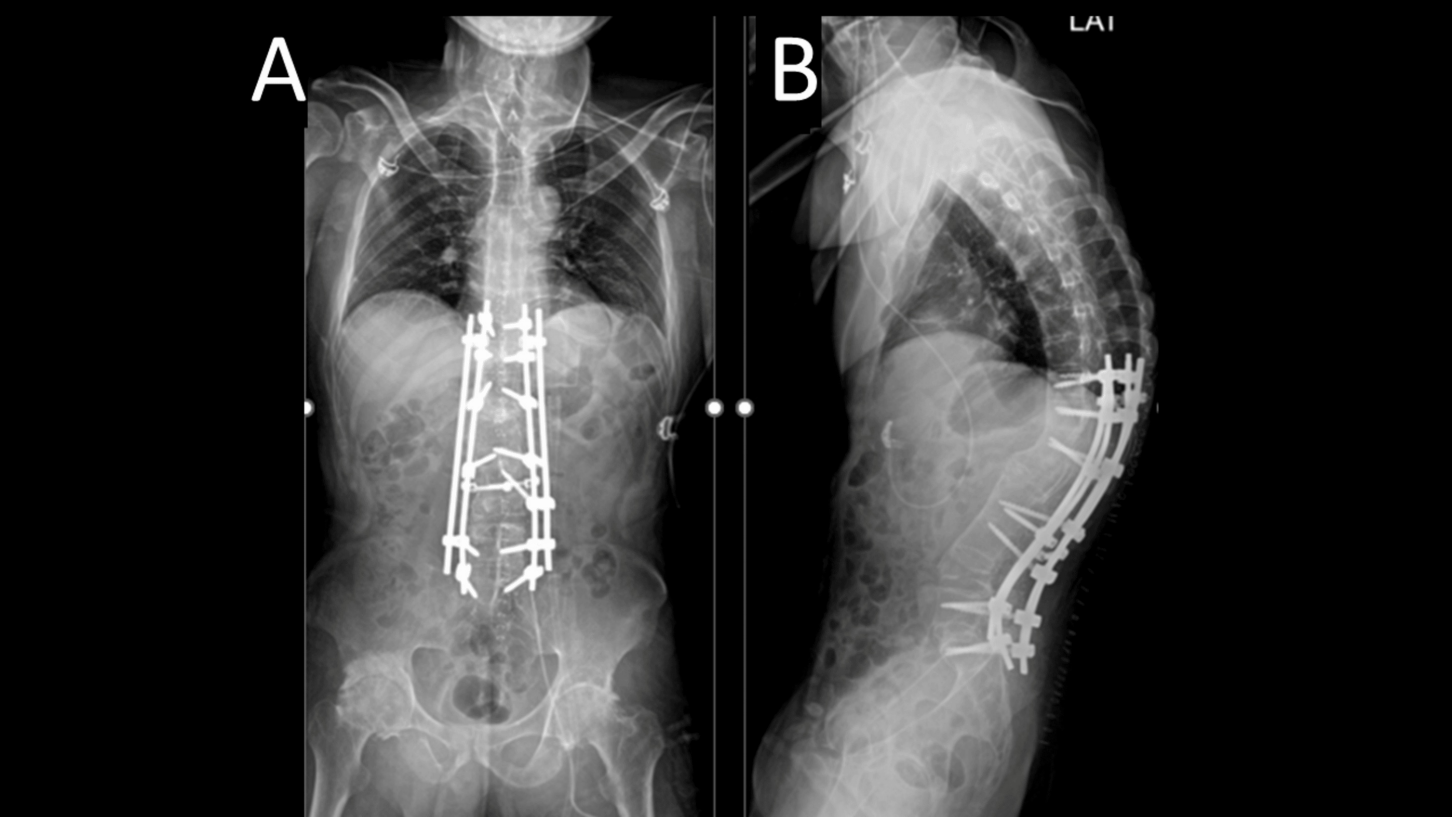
Postoperative X-ray of the patient Figure 6A: Postoperative X-ray (anteroposterior view) showing posterior instrumentation and fusion done from T10-S1; Figure 6B: Postoperative X-ray (lateral view) showing posterior instrumentation and fusion done from T10-S1, showing 70 degrees of kyphosis corrected
